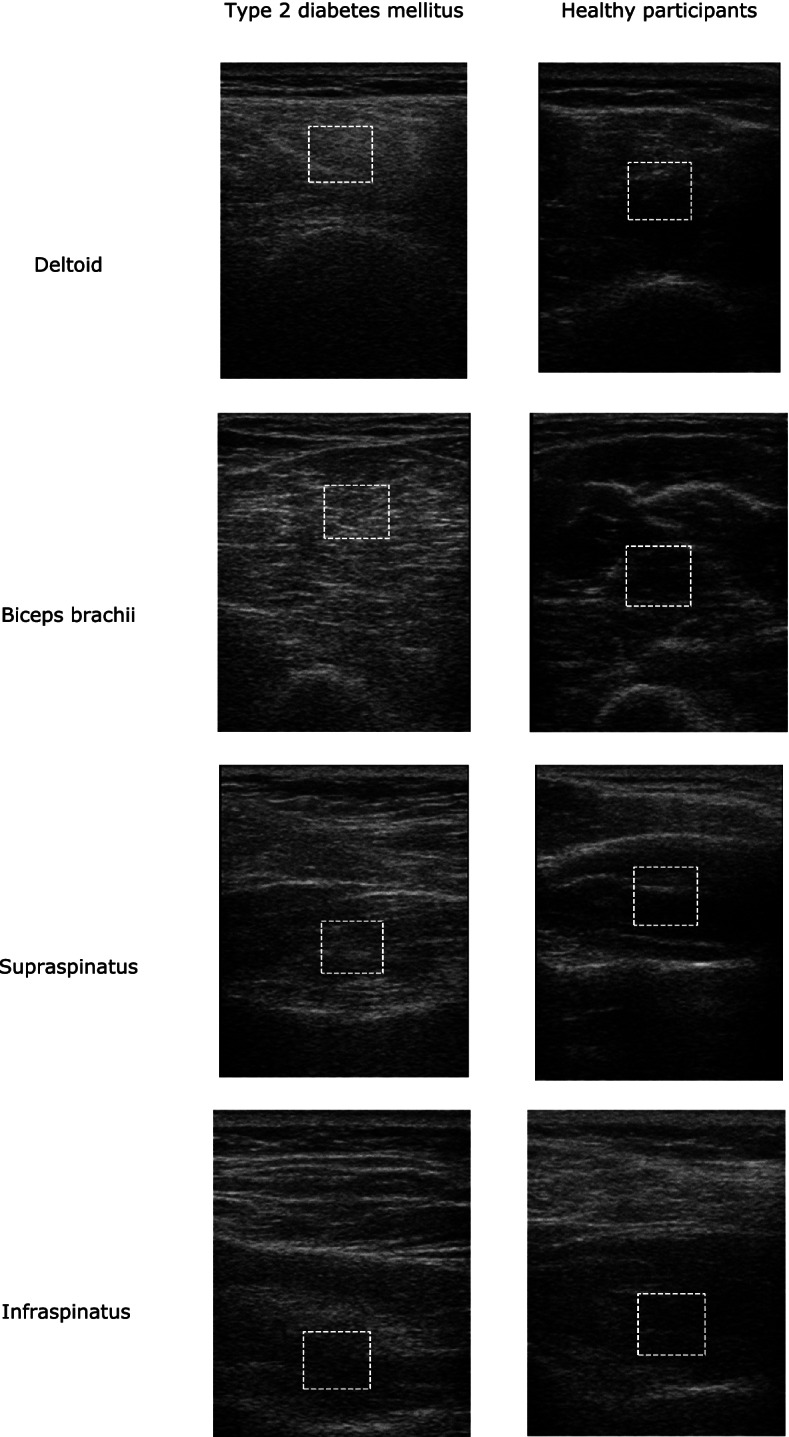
Muscle ultrasound images of patients with type 2 diabetes mellitus having shoulder pain and healthy participants. Compared with normal muscles, patients with type 2 diabetes mellitus have muscles characterized by a higher echogenicity (whiter appearance). The white dashed lines delineating the region of interest were manually chosen for analysis
